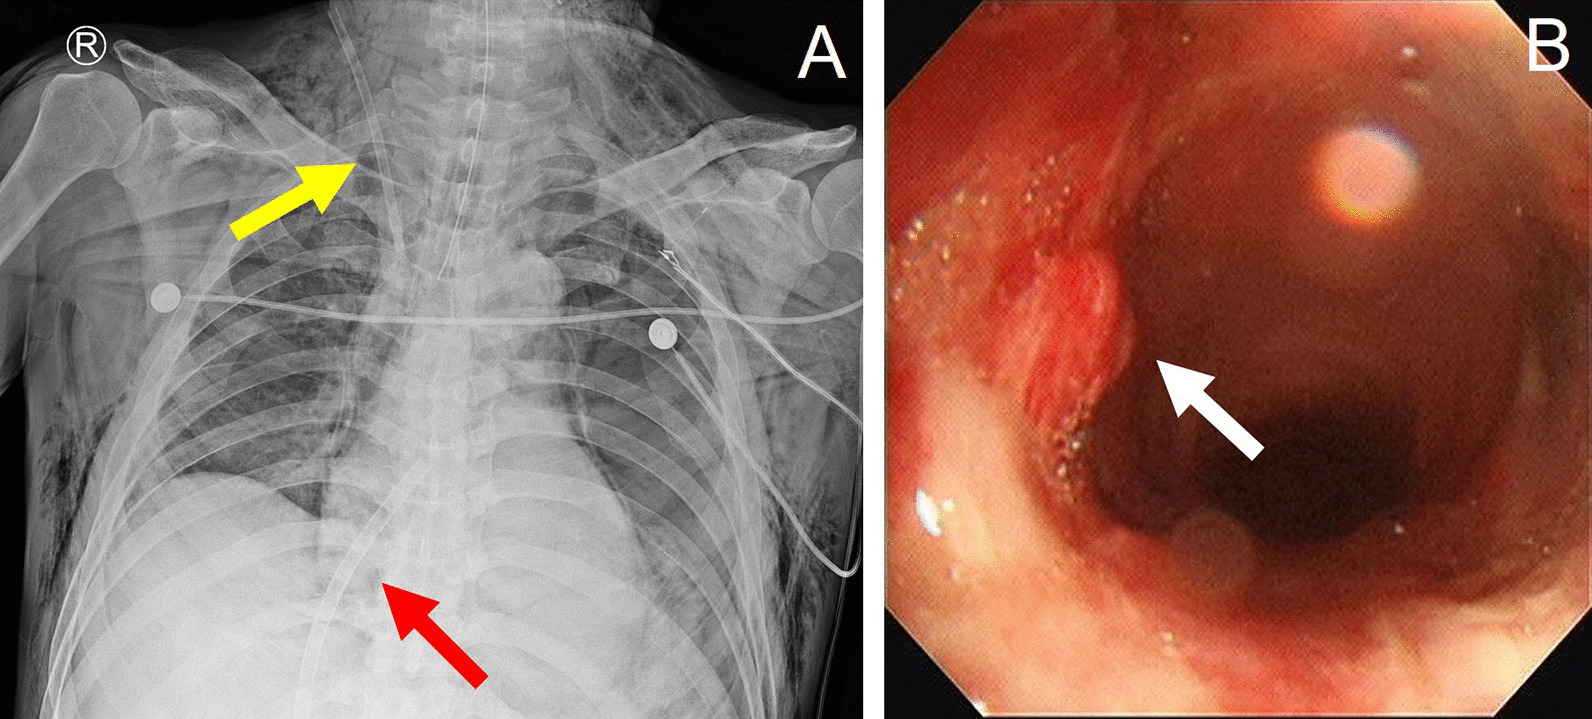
Postoperative finding. a Postoperative X-ray; yellow arrow: an advanced venous access catheter. (Arrow multi-lumen access catheter 9Fr, Teleflex, USA), ARROW® MAC (Multi-Lumen Access Catheter) red arrow: ECMO drain catheter. (MAQUET PLS, NJ, USA). b On the third day after surgery, bronchoscopy bronchoscopic finding. White arrow: injury site
